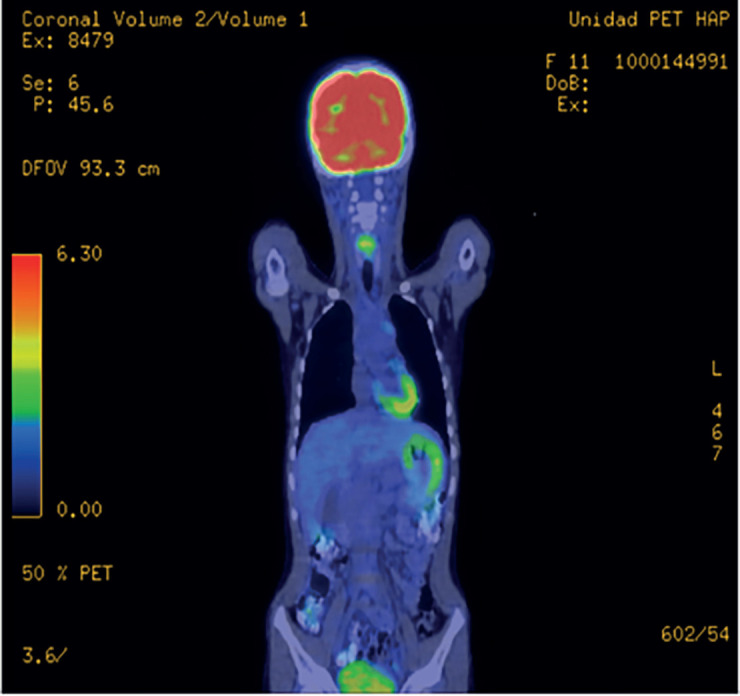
Coronal PET CT. Source: Clinical record.
